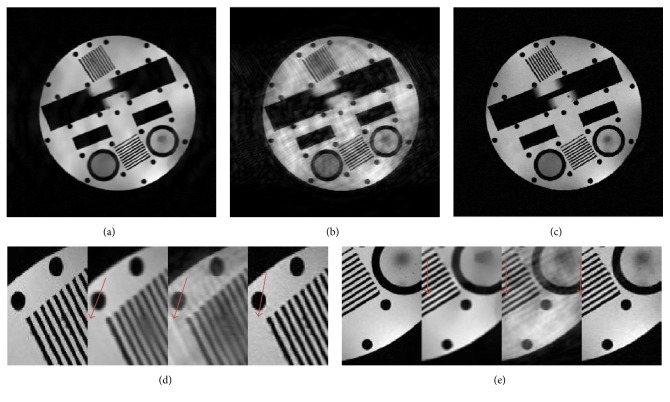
Comparison of reconstruction of a physical MR image with noise of *σ* = 30. ((a), (b), (c)) Reconstruction results of DLMRI, SHTGV, and DLTGV at 8-fold undersampling. ((d), (e)) The area of enlargements corresponding to (a), (b), and (c).
